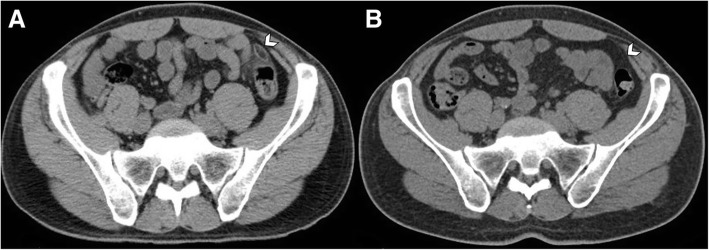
Evolution of acute epiploic appendagitis in a 37-year-old man. a Axial non-contrast CT image shows an inflamed fatty ovoid lesion near the sigmoid colon (arrowhead), with hyperattenuating ring sign and nearby fat stranding. b CT scan, performed 1 year later for other reasons, demonstrates complete resolution of imaging findings of epiploic appendagitis (arrowhead)
